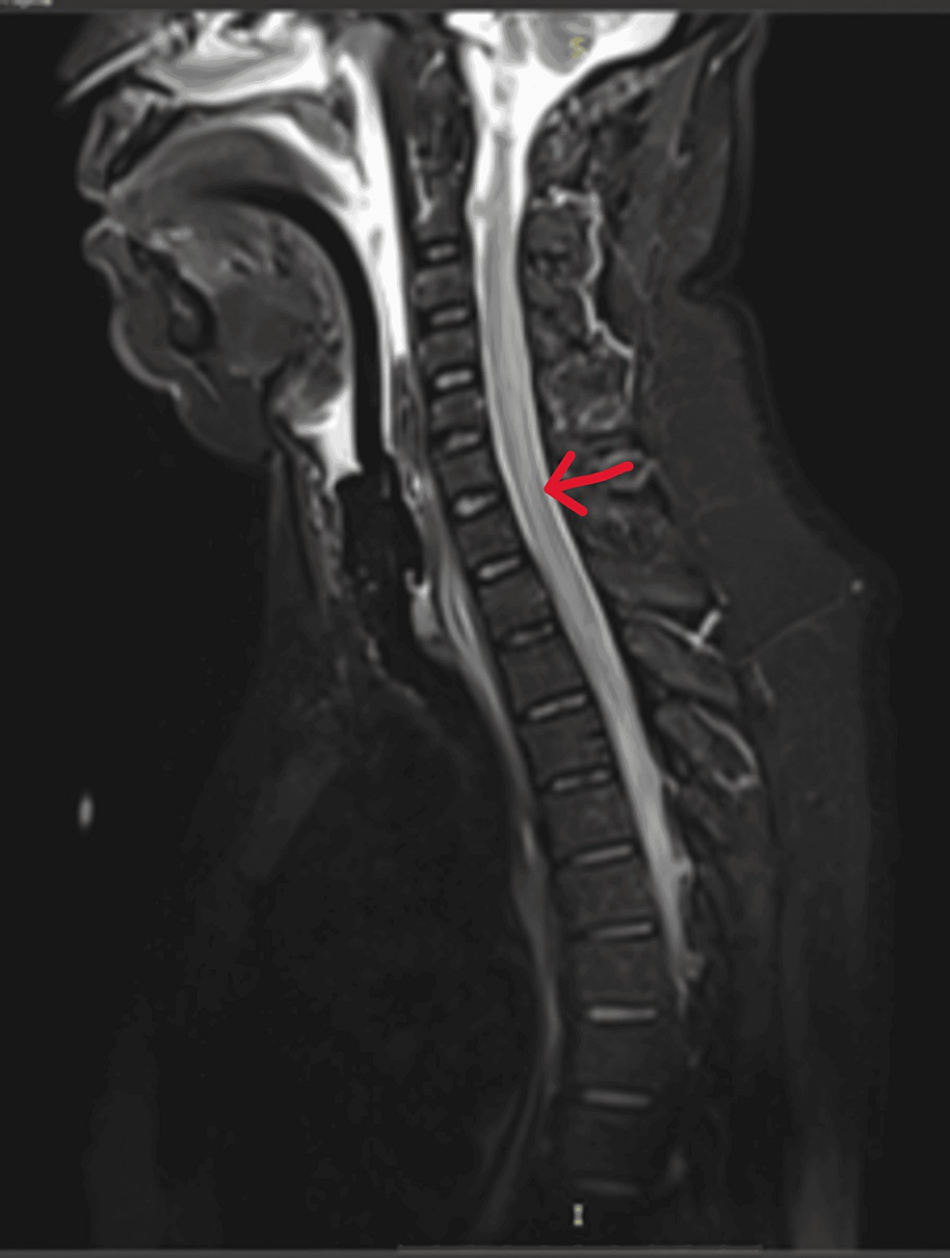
T2-weighted MRI of the cervical and thoracic spine (sagittal section) showing long segment intramedullary high signal intensities. MRI: magnetic resonance imaging
